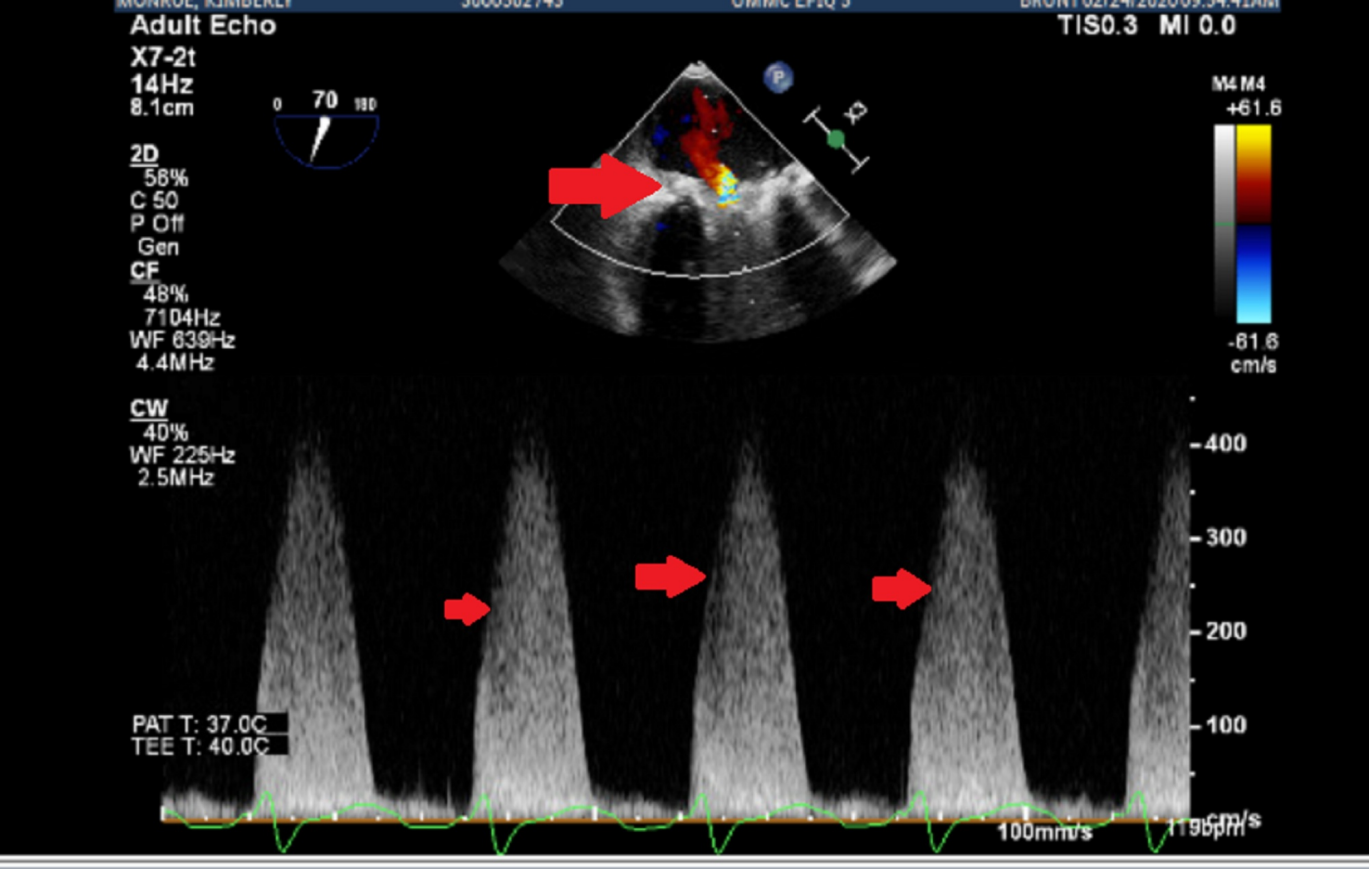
Transesophageal echocardiogram with severe mitral stenosis with elevated gradients across the prosthetic mitral valve
